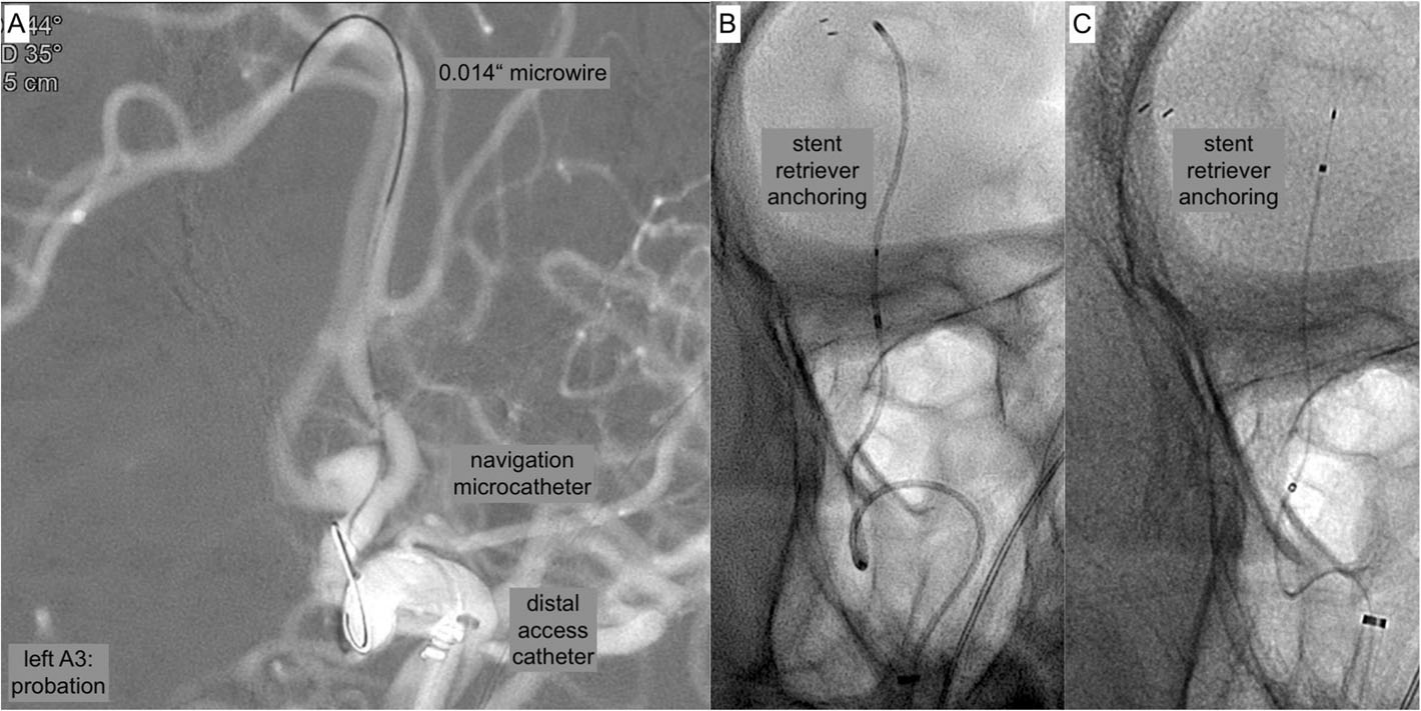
shows representative images of the probation with the 0.0165″ microcatheter and subsequent anchoring of the pREset, preparing the exchange maneuver of the stiffer and less versatile large bore microcatheter required for FDS implantation. a Roadmap demonstrating probation of the left handed A3 segment. b Plain radiography showing the microcatheter of the initial step and its spatial relation to the vascular anatomy in working projection. c Plain radiography showing the microcatheter of the initial step and its spatial relation to the vascular anatomy in a supplementing lateral projection
